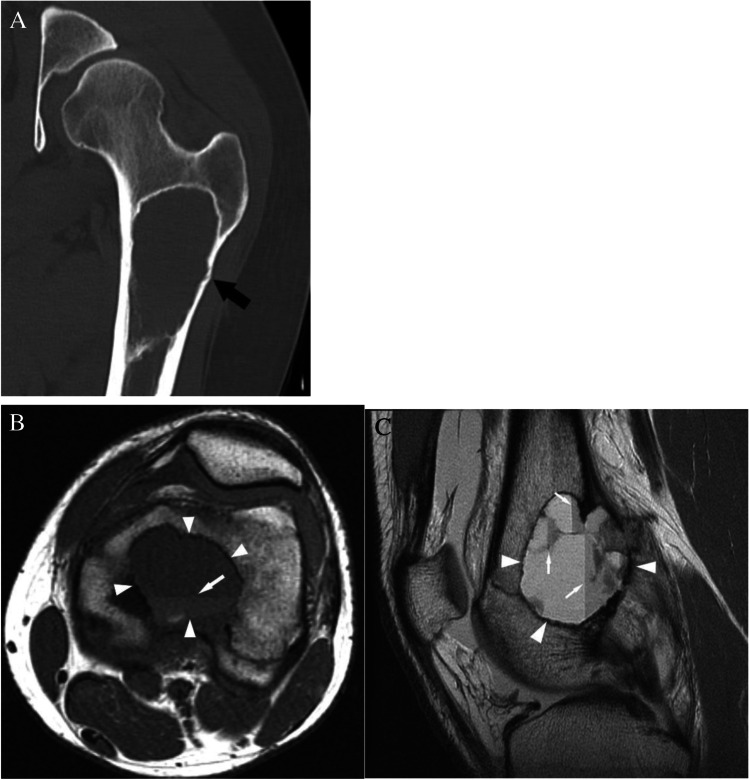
Case 7. A 27-year-old female presents with vague thigh pain for 1 year. Coronal CT image reveals a solitary focal lucent lesion in the left proximal femoral metaphysis with a well-defined sclerotic margin, cortical involvement (arrow), and mean HU of 18 units; a simple bone cyst at surgery. B and C 18-year-old female presents with knee pain worse over last 3 months. MRI of the left knee reveals a cystic lesion (arrowheads) involving the distal femoral metaphysis with fluid levels (thin arrows) due to internal blood products. The lesion has a well-defined sclerotic margin and demonstrates mild expansile remodeling. The lesion was aneurysmal bone cyst at treatment
